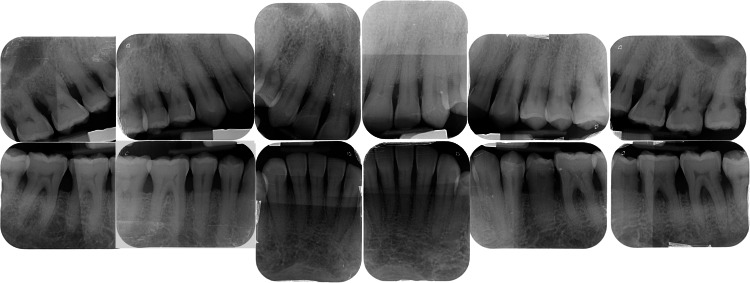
Case 2: initial radiographs. Note the presence of generalized bone loss.
